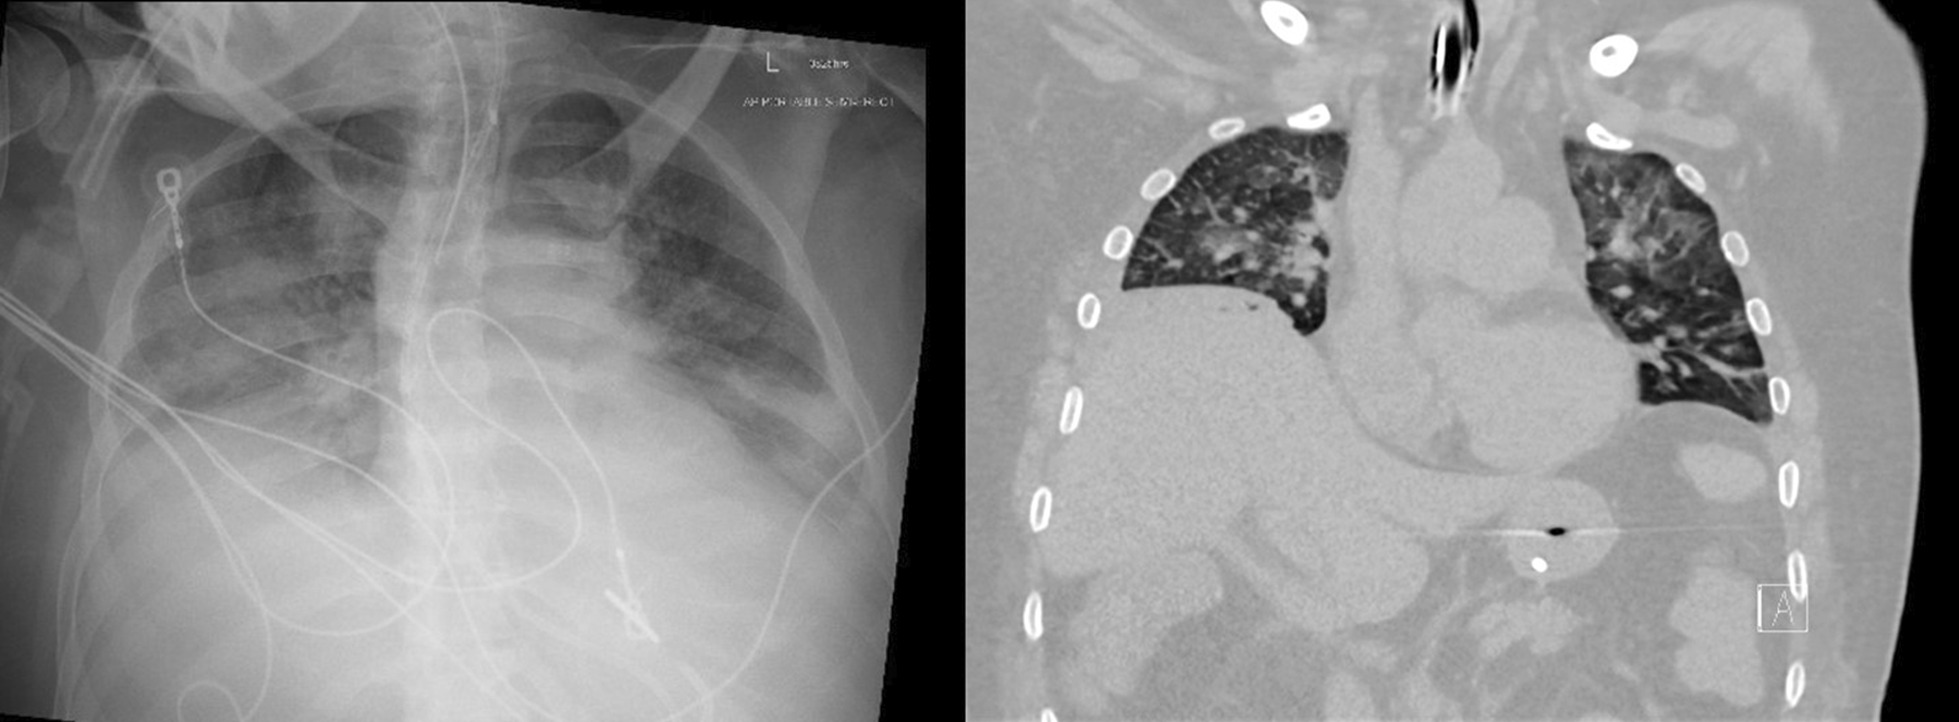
Chest radiograph (left panel) and chest CT (right panel) in a patient with myotonic dystrophy and respiratory failure. Bilateral hemidiaphragm elevation (right hemidiaphragm elevation is greater than that of the left) likely caused by diaphragm myopathy
